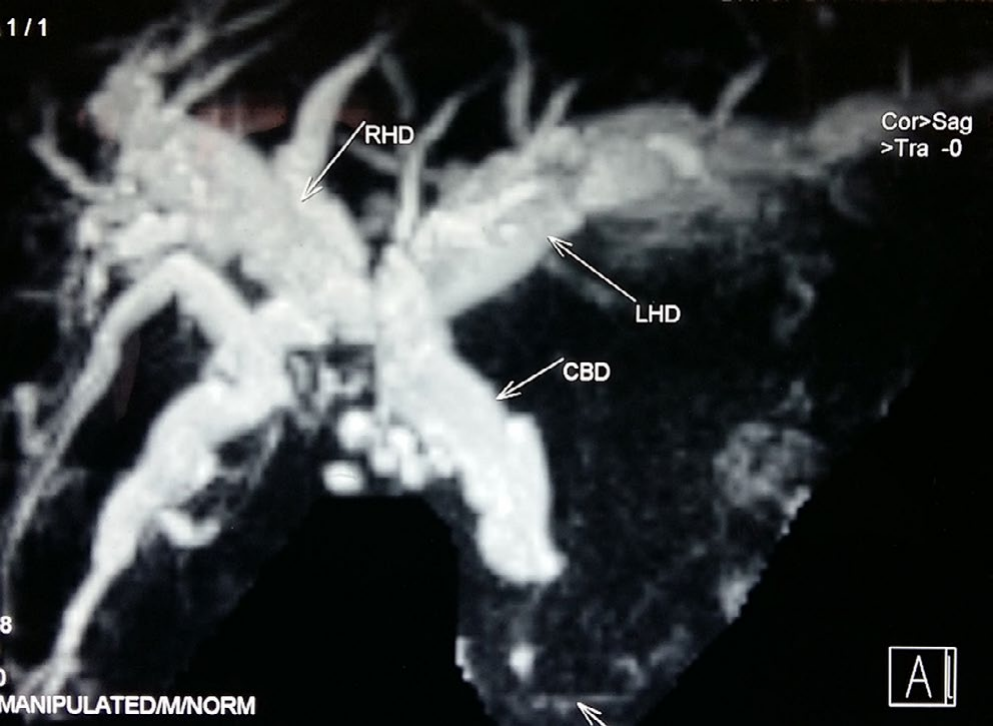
T2 Magnetic resonance imaging showing long segment distal common bile duct stricture with proximal biliary dilatation due to the portal biliopathy
